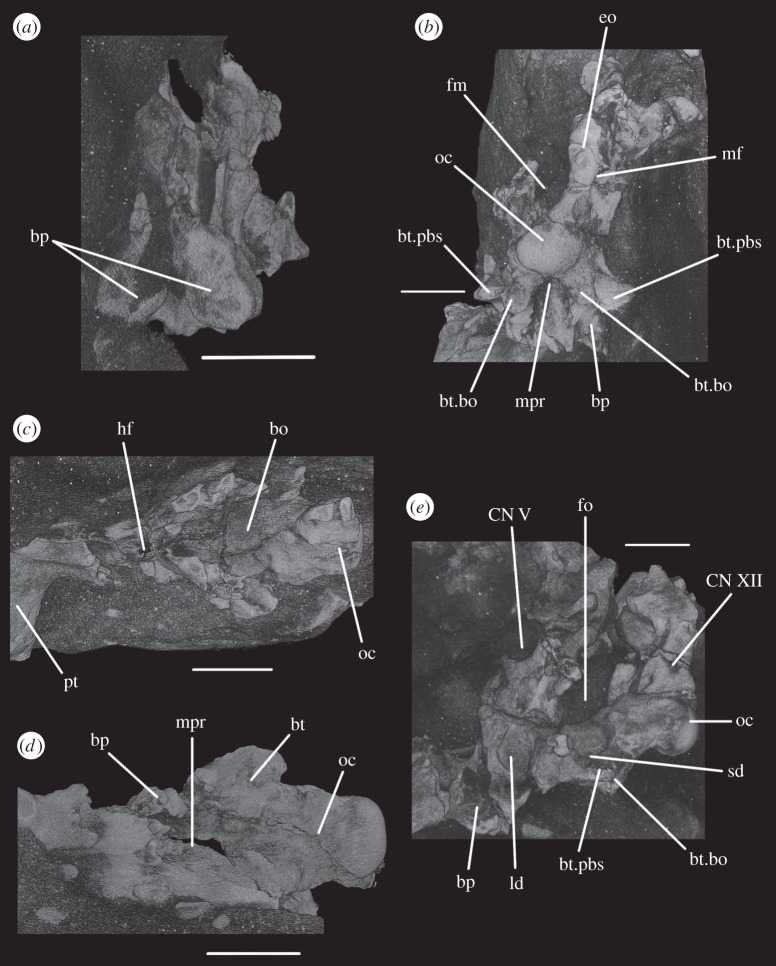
CT reconstructions of braincase of UMZC T.692 in (*a*) anterior, (*b*) posterior, (*c*) dorsal (in cross section to expose braincase floor), (*d*) ventral and (*e*) left lateral views. For abbreviations, see table 1.
